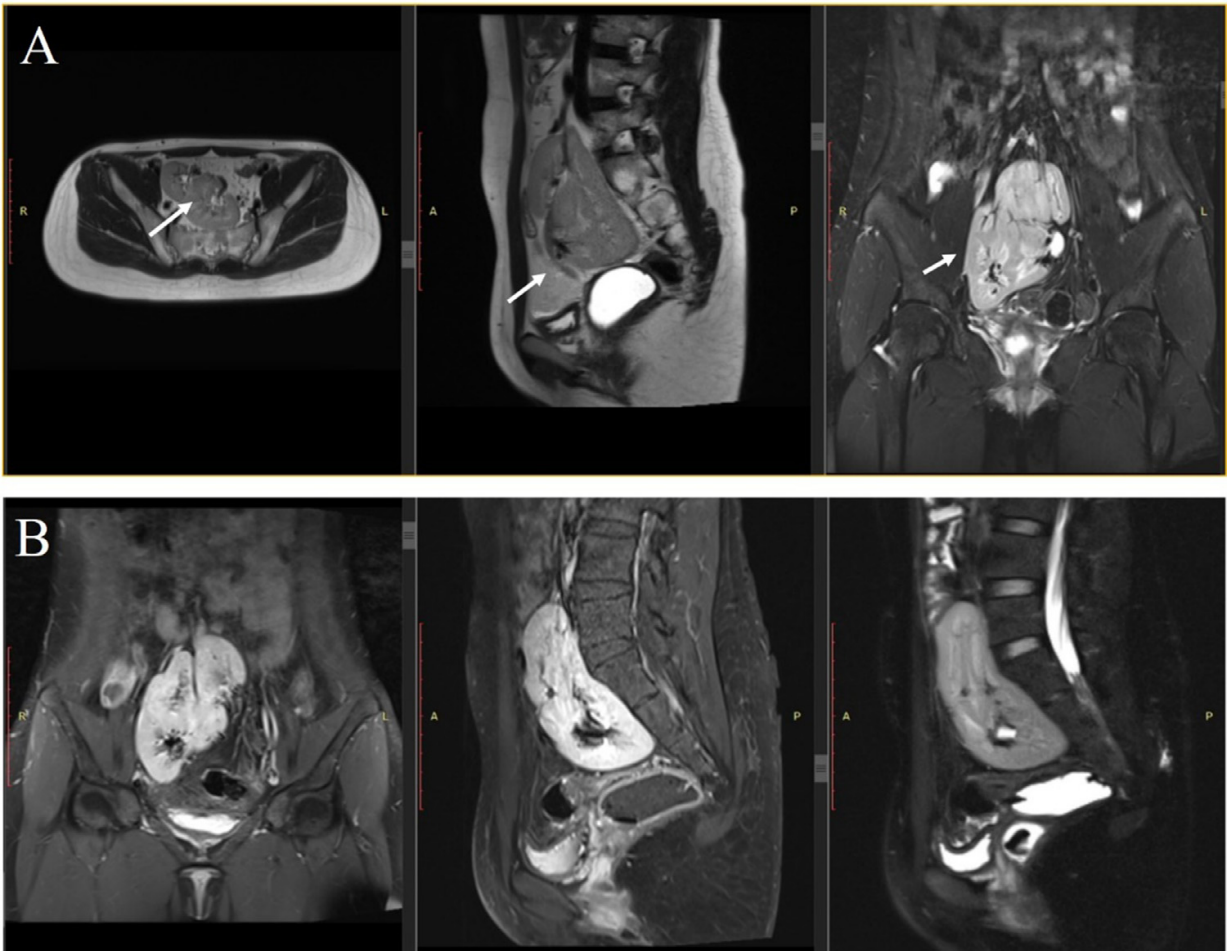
Contrast MRI (A) normal uterus and adnexa were not found on T2FS. Ectopic kidney was seen within the right pelvic cavity (white arrow). (B) Similar finding was found on T1W1.
